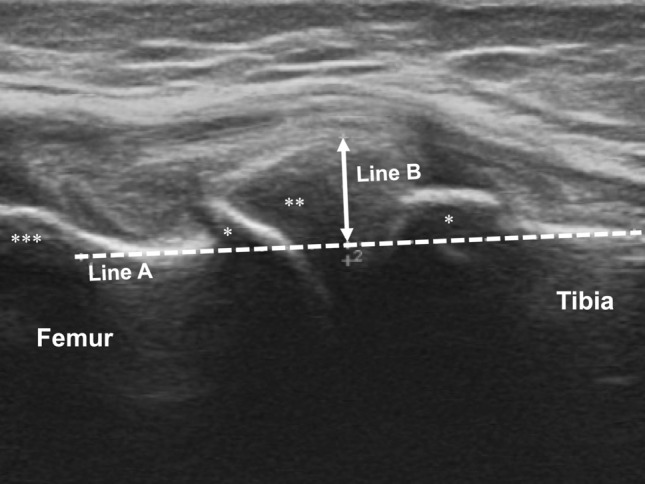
Ultrasonographic evaluation of medial meniscus extrusion. *Osteophyte, Medial meniscus, *Medial femoral epicondyle. Line A was drawn to connect the cortex of both the femur and tibia, thereby tracing the femoral cortex at the bottom of the medial femoral epicondyle. Line B was drawn perpendicularly from the bottom of Line A to the most medially extruded part of the medial meniscus. Line A was drawn through the osteophyte bases to avoid the bony interference of osteophytes throughout the length of Line B. Finally, the length of Line B (mm) was measured as the medial meniscus extrusion_._
